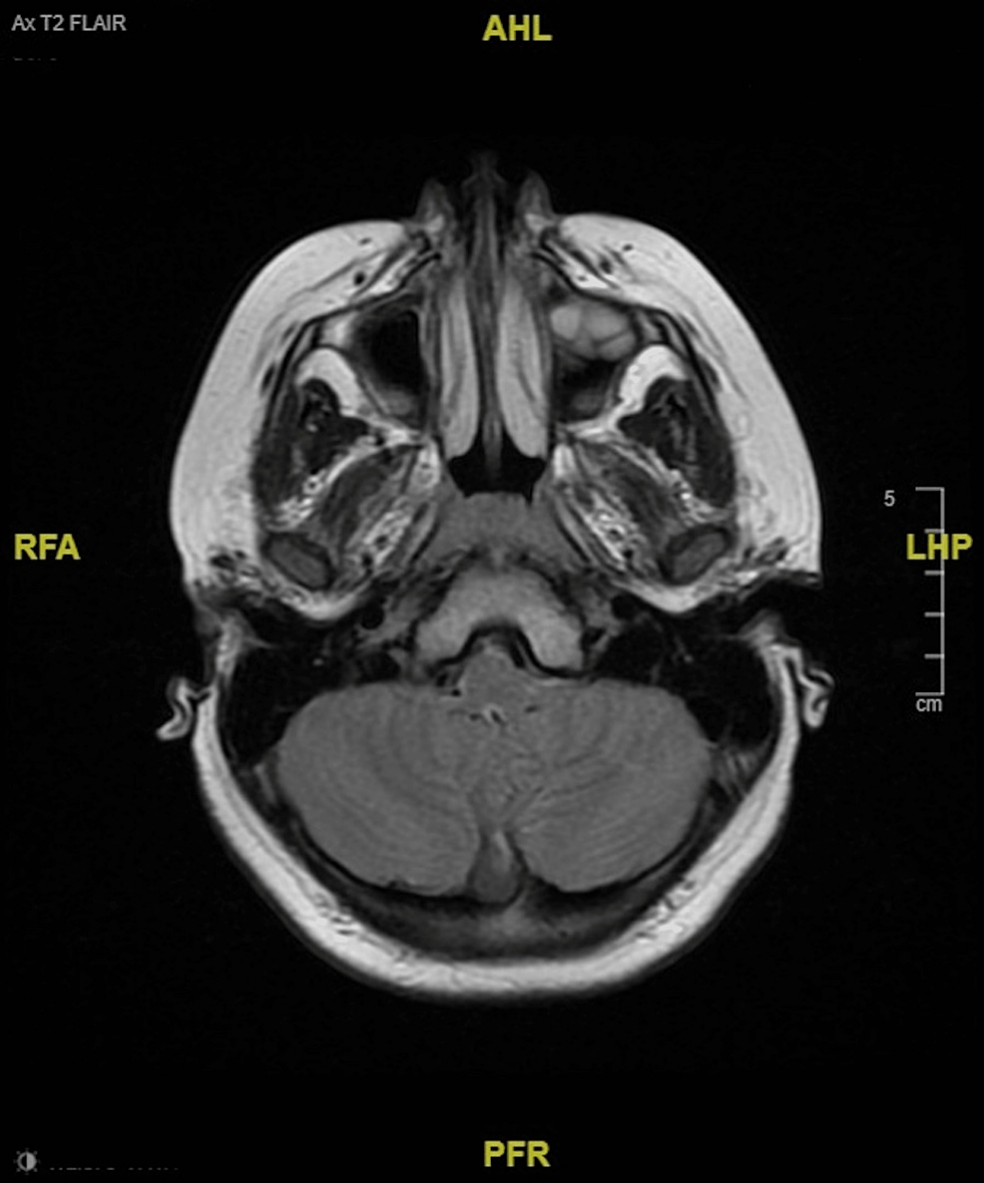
MRI brain shows normal cerebellum The image shows a T2-weighted FLAIR sequence taken on a 1.5-Tesla scanner. Dotarem intravenous contrast (12 mL) was administered. The cerebellar hemispheres have normal volume without evidence of signal change or mass effect. FLAIR: Fluid-attenuated inversion recovery.
